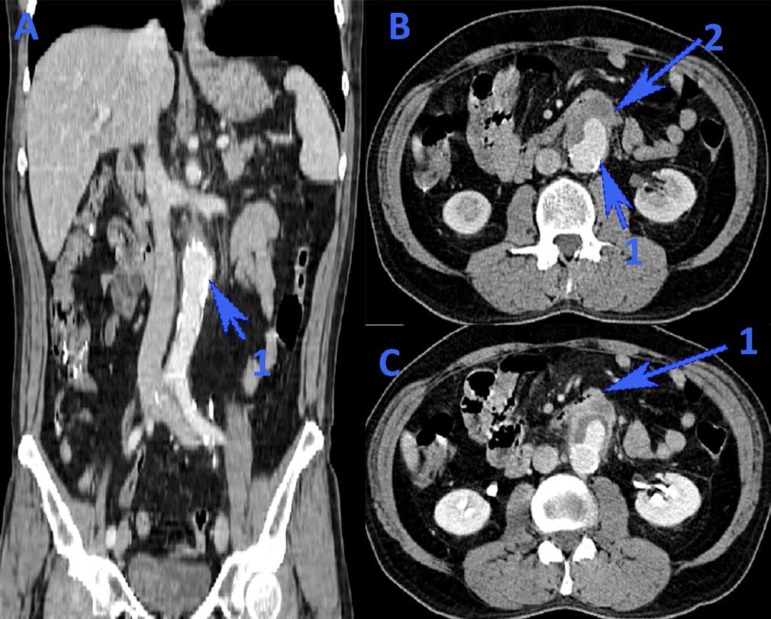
Computed tomography images. A – Coronal view showing the infrarenal
